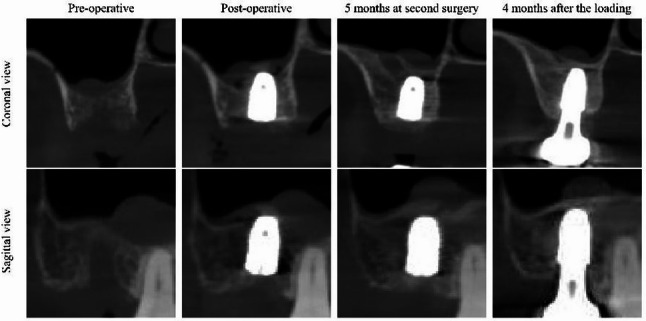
Representative radiographic image showing extensive bone formation at the implant apex. Cross-sectional CBCT view demonstrates that new bone has formed beyond the original grafted DDM area, completely covering and integrating over the graft particles
